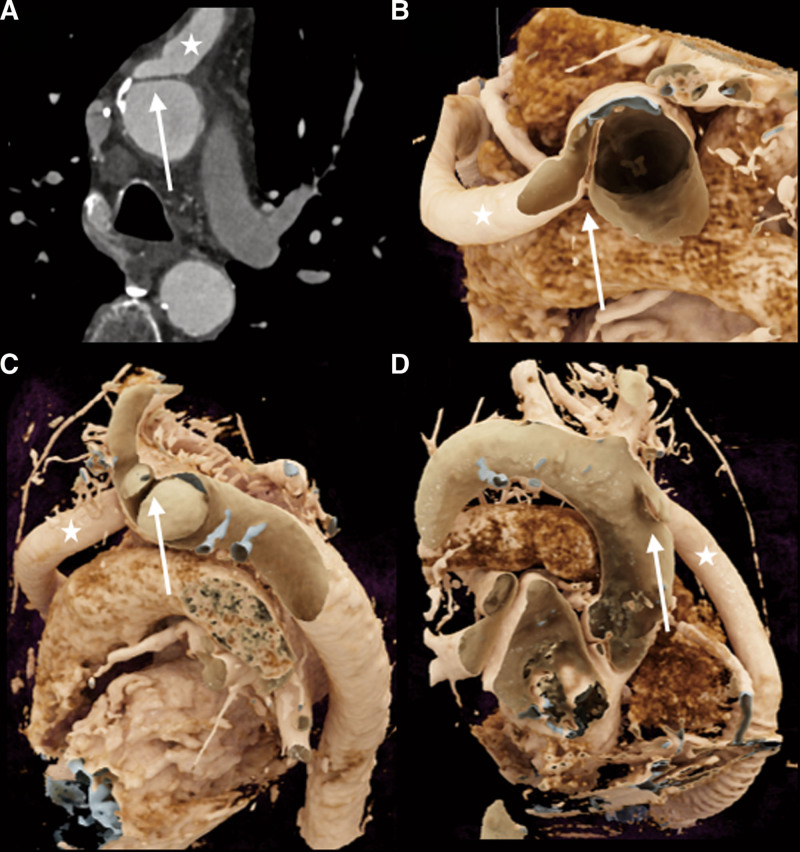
A cardiac computed tomography angiogram. A–D: chronic dissection (arrow) of the ascending aorta, directly in front of the orifice of the LVAD outflow graft (asterisk). LVAD, left ventricle assist device.
